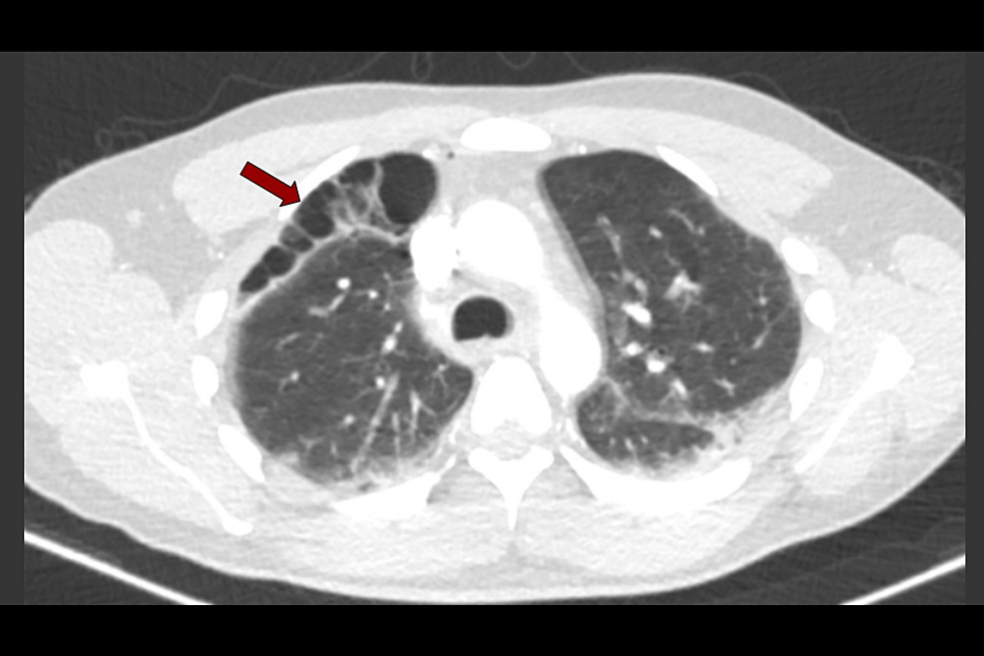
CTPA day 9: axial slice showing the location of subpleural bullae anteriorly (red arrow) CTPA, computed tomography pulmonary angiogram
